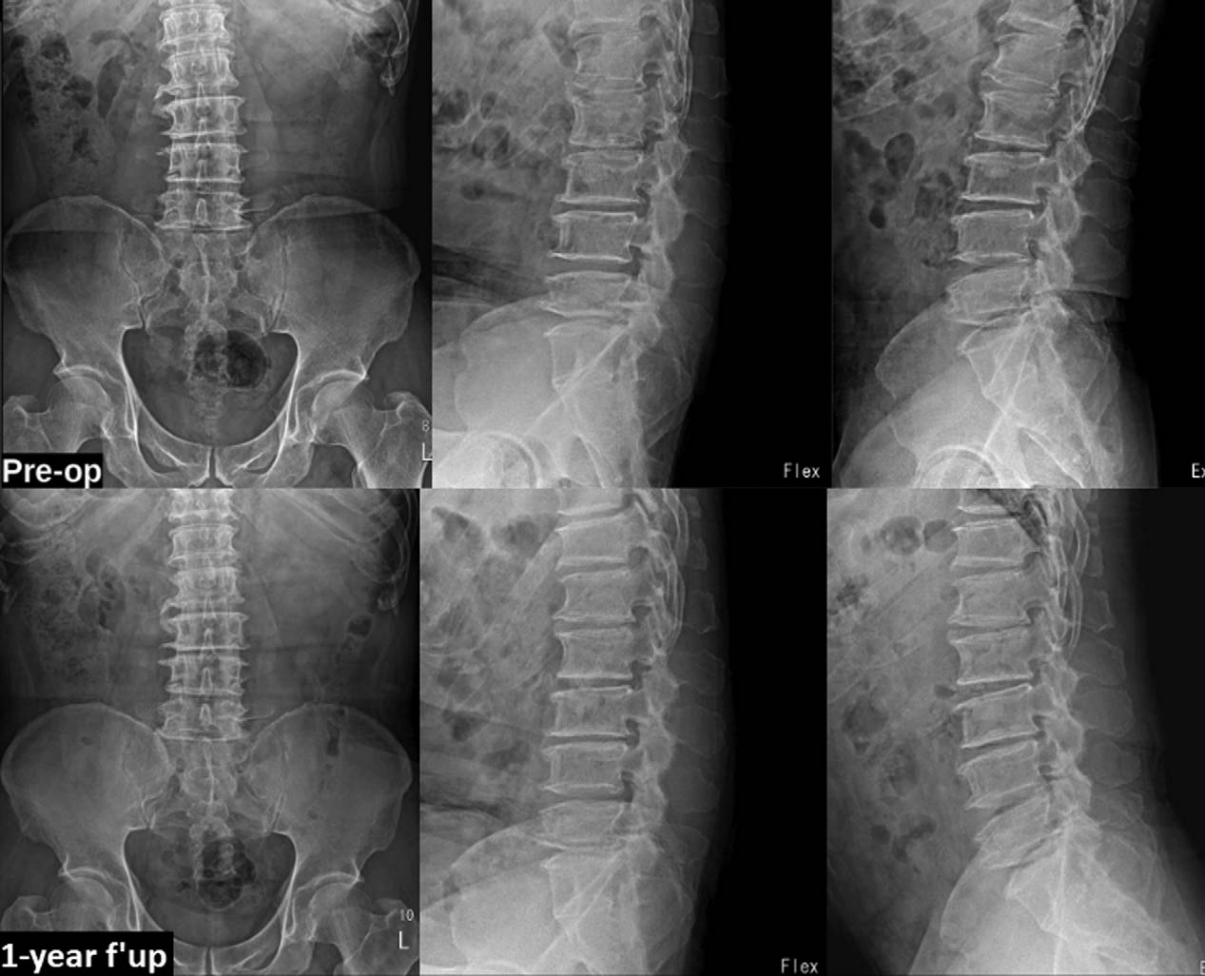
X-rays of the same patient comparing pre-operative and at 1-year follow-up shows no aggravation of instability.
